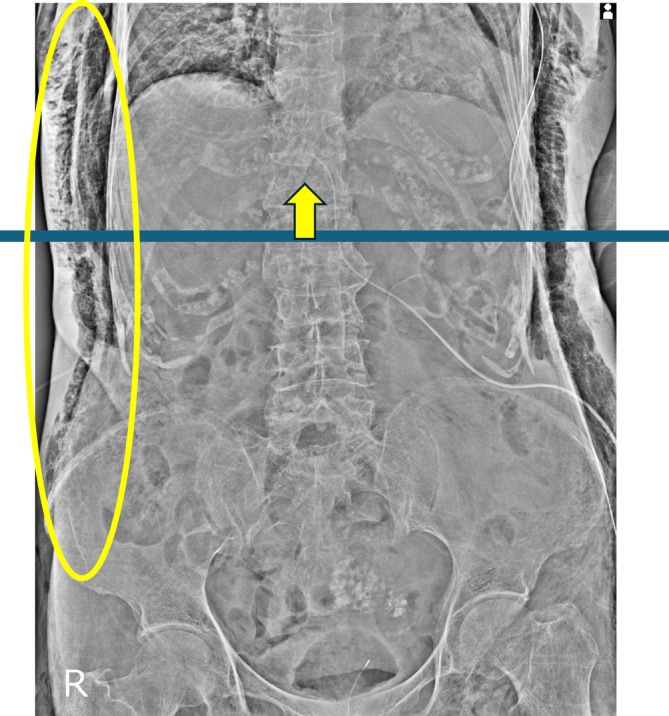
Representative postoperative abdominal radiograph of an SE‐positive case. Subcutaneous emphysema extends cranially beyond the level of T12, with gas tracking into the lower thoracic wall above the horizontal line. The arrows indicate the approximate level of T12 and the maximal cranial extent of subcutaneous emphysema.
